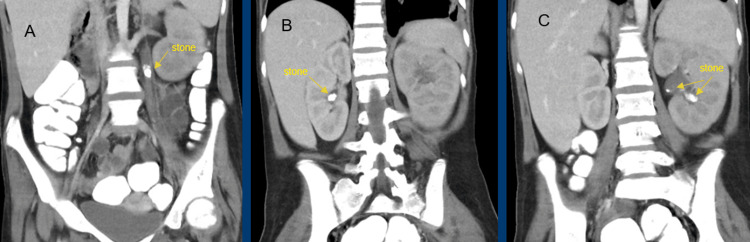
Initial CT with bilateral ureteral calculi and left hydronephrosis A) Left proximal ureteral stone. B) Right kidney stone. C) Left lower calyx stone and another one in the ureteral-pyelo junction.
